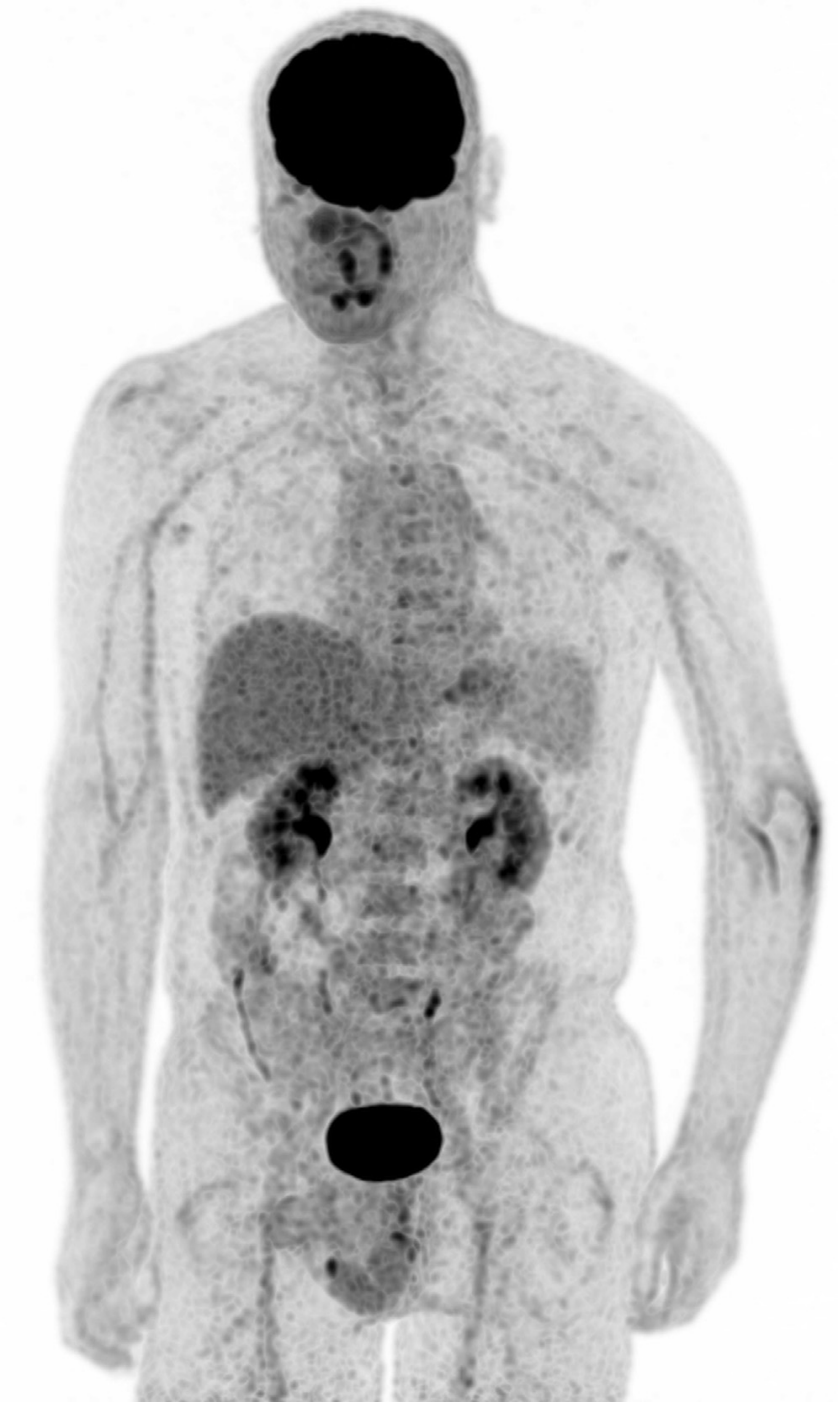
Technetium FDG PET/CT scan at 2.5 years postoperation.
